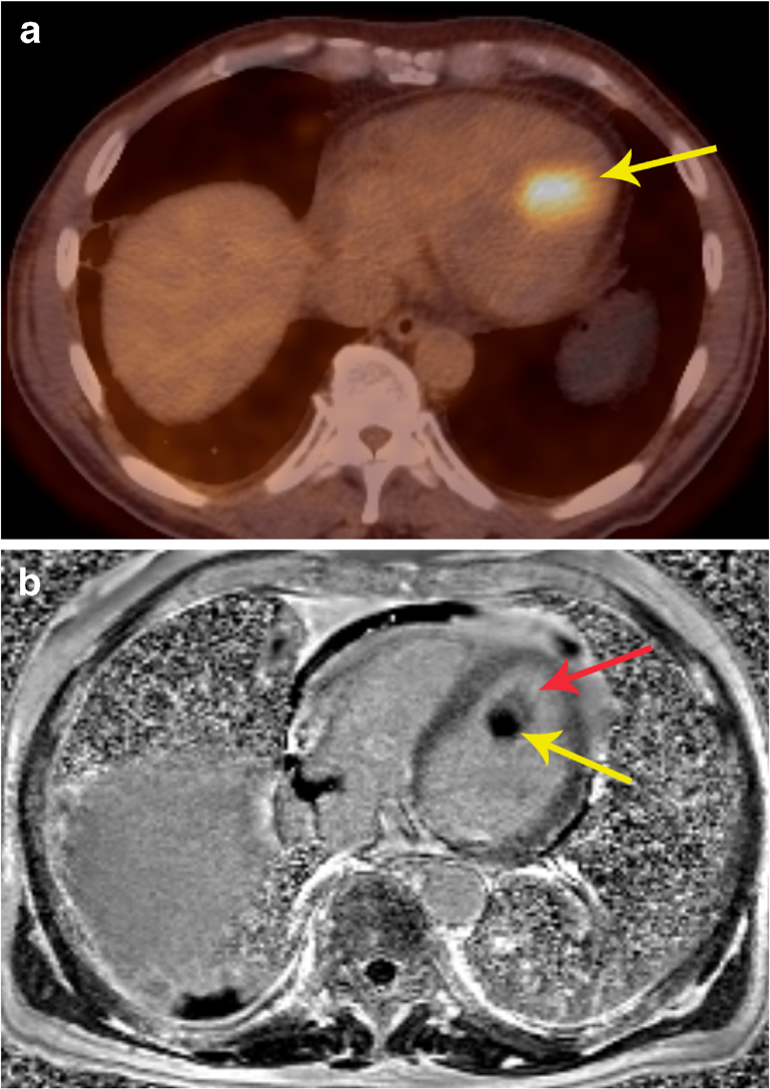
Characterization of mass. a Axial FDG-PET/CT scan in a patient with known thyroid cancer showed intensely hypermetabolic lesion (arrow) in the heart, which could not be localized clearly. b Four-chamber delayed enhancement cardiac MRI shows the mass to be entirely located within the left ventricular cavity. The mass has two distinct components, an enhancing component (red arrow) and a non-enhancing component (yellow arrow), which correspond to metastatic lesion and superimposed and bland thrombus, respectively. The patient was placed on anticoagulants in addition to chemotherapy
